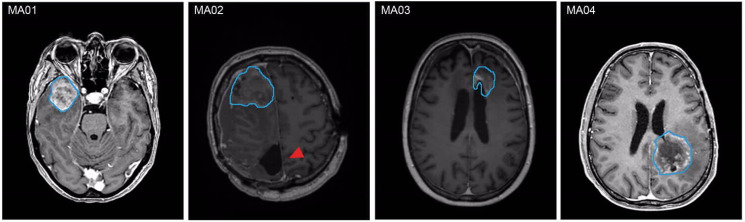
MRI characteristics of the patients included in this study. MRIs show contrast-enhanced, suspected recurrent high-grade glioma prior to re-resection. Outlined in blue is the resection cavity after the second surgical treatment with partial or complete resection of the contrast-enhanced tumor. In the MRI of patient MA02, the resection cavity from initial surgery is marked with a red arrowhead; in this case, the plane of the resection cavity is within the same axial plane as the recurrent contrast enhancement.
